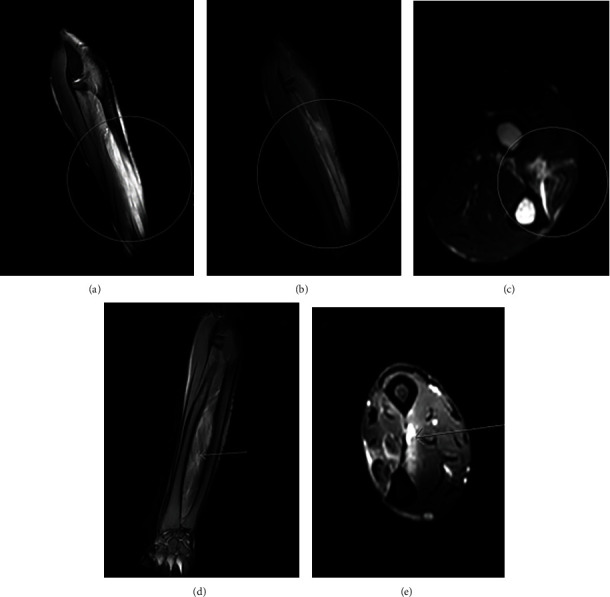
Magnetic resonance imaging of the arms. (a)–(c) Heterogenous T2-hyperintensitivity of the extensor carpi ulnaris muscle of the left arm, with surrounding edema of the fascia and subcutis.(d, e) Heterogenous T2-hyperintensitivity of the deep flexor and extensor muscles, superficial extensor muscles, and extensor carpi radialis brevis muscle. Edema of the fascia was also observed.
